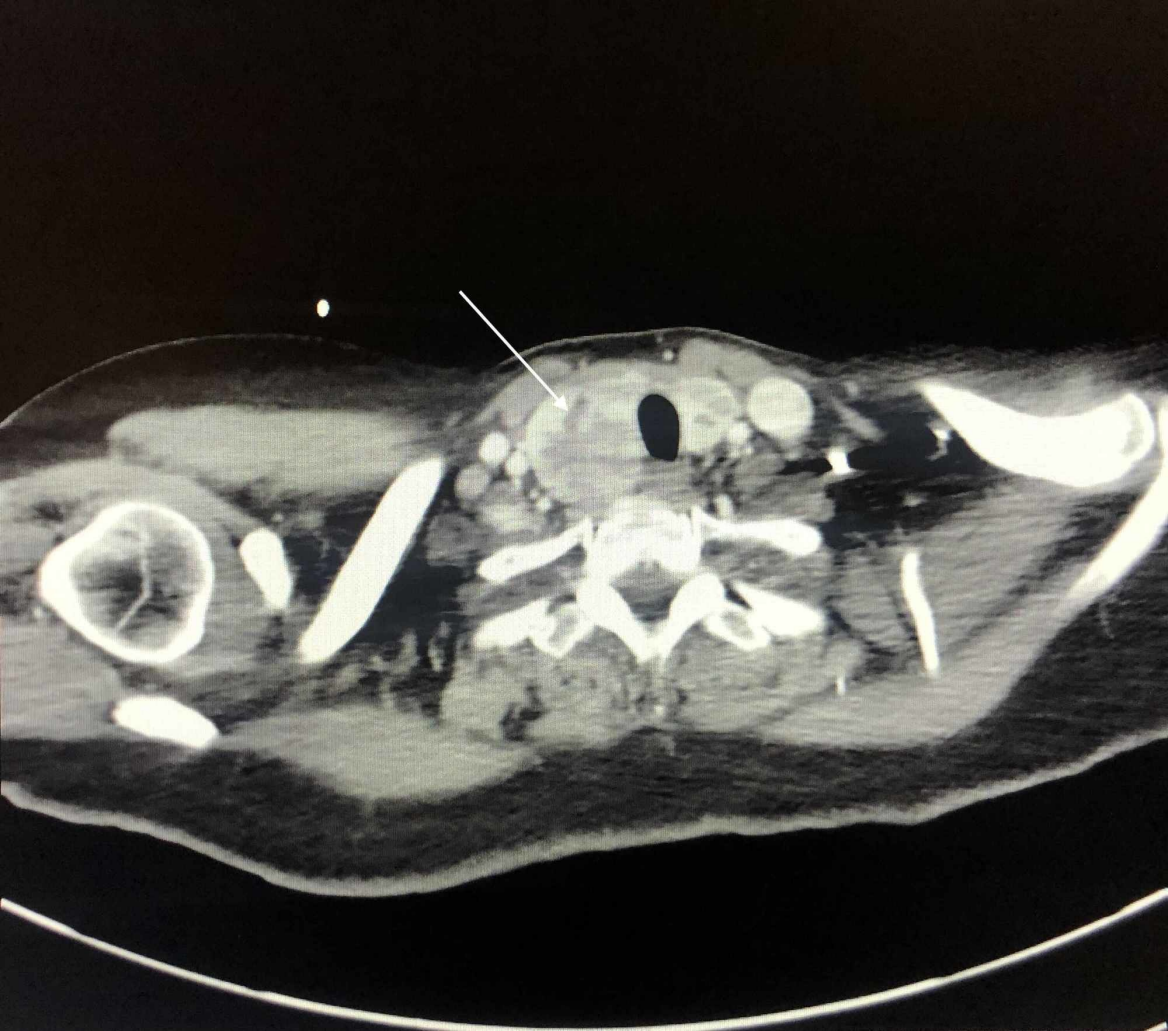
Chest CT shows right thyroid nodule.
